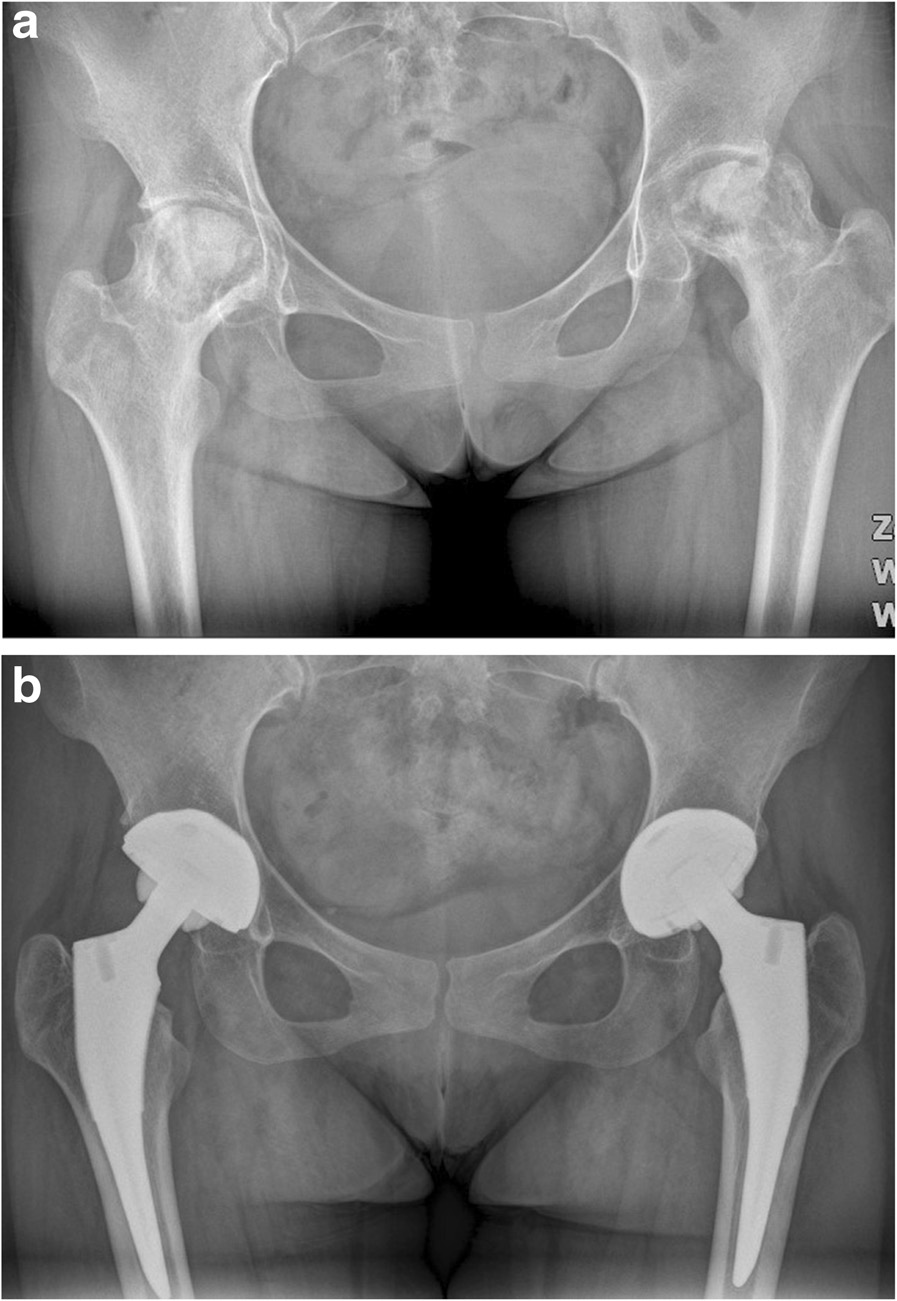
a A preoperative radiograph of the hip of a 73-year-old woman who had bilateral femoral head osteonecrosis. b A radiograph at 40 months in the right hip and 43 months in the left hip after implantation of short cementless femoral stems showed solid fixation in a satisfactory position
